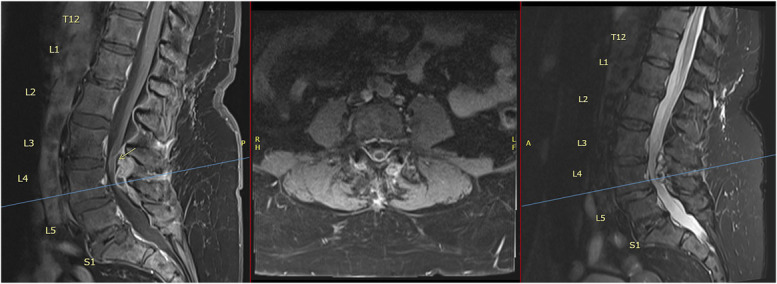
Preoperative MRI—Case 1. The sagittal (left) and axial (center) T1-weighted MRI with contrast and sagittal T2 (right) demonstrate the presence of a posterior epidural lesion at the L3-4 level (yellow arrow) and L4-5 levels with significant compression of the thecal sac and moderate to severe spinal canal narrowing. The axial T1-weighted image with contrast (center) shows a facet joint-associated posterolateral epidural lesion corresponding to the synovial cyst, with peripheral contrast enhancement.
